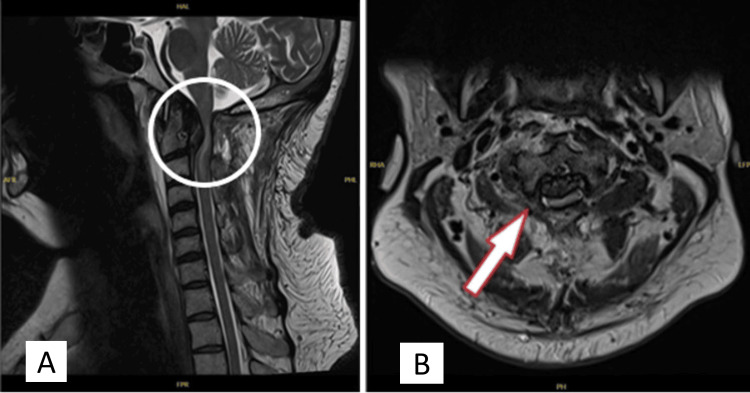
Follow-up (2 months post-decompression) MRI of the cervical spine with axial (A) and sagittal views (B) showed spinal cord compression and interval progression of the intramedullary signal hyperintensity of the cord (indicated by circle and arrow, respectively).
